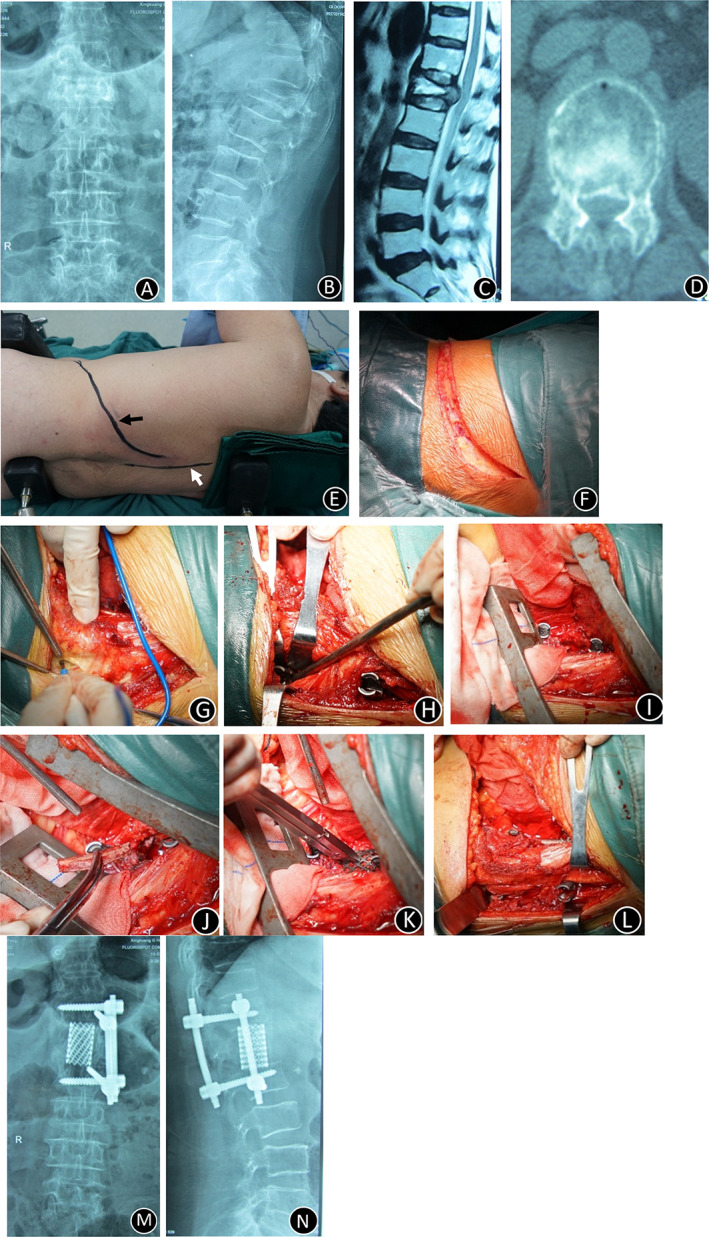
Clinical imaging from one representative patient (73‐year‐old woman) who complained of a lumbar 1 compression fracture. (A, B) X‐ray images of lumbar vertebrae anteroposterior and lateral position X‐ray films showed an L1 vertebral compression fracture. (C) T2‐weighted sagittal preoperative MRI of the lumbar spine showed that the spinal cord was compression by bone fragments. (D) The lumbar vertebrae CT indicates that the bone fragment had burst into the vertebral canal. (E) The patient remained in the lateral decubitus position during the operation; note the mark of the incision (black arrow) and the spinous process (white arrow). (F) An oblique incision was made along corresponding ribs. (G) After the anterior decompression was completed, the entry points of the posterior pedicle screws were exposed through the unilateral paraspinal muscle approach under the same incision. (H) The posterior pedicle screws were inserted into the vertebral pedicles adjacent to the fracture vertebra. (I) Two pedicle screws were inserted into the lateral side of the proximal and distal vertebras. (J) A rib graft was placed between the proximal and distal vertebras of the fractured vertebra. (K) An anterior metal cage filled with cancellous bone chips was implanted between the proximal and distal vertebras of the fractured vertebra. (L) Anterior fixation and posterior unilateral pedicle screw fixation is shown under one approach. (M,N) The postoperative X‐ray imaging indicated that the location of the internal fixator was excellent.
